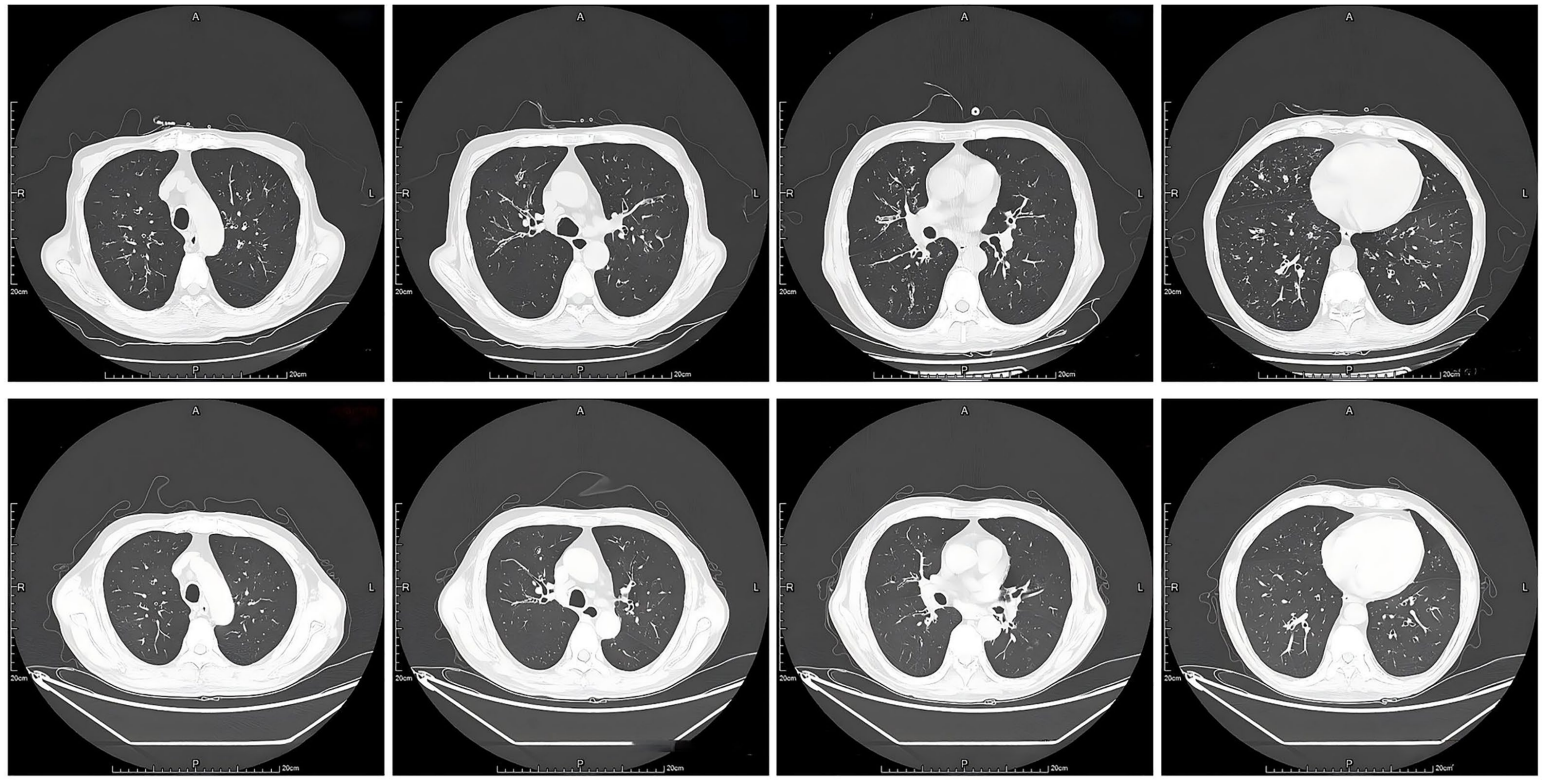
Chest CT scan. Top, chest CT scan in September 2024; bottom, chest CT scan in February 2025.
